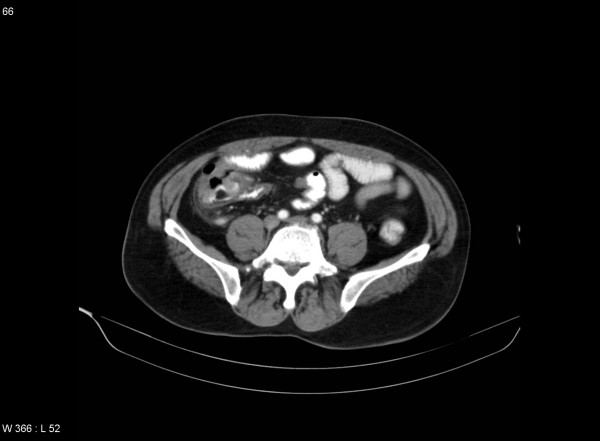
CT scan of the abdomen showing a polypoid mass of the cecum that almost completely obstructs the bowel lumen.
